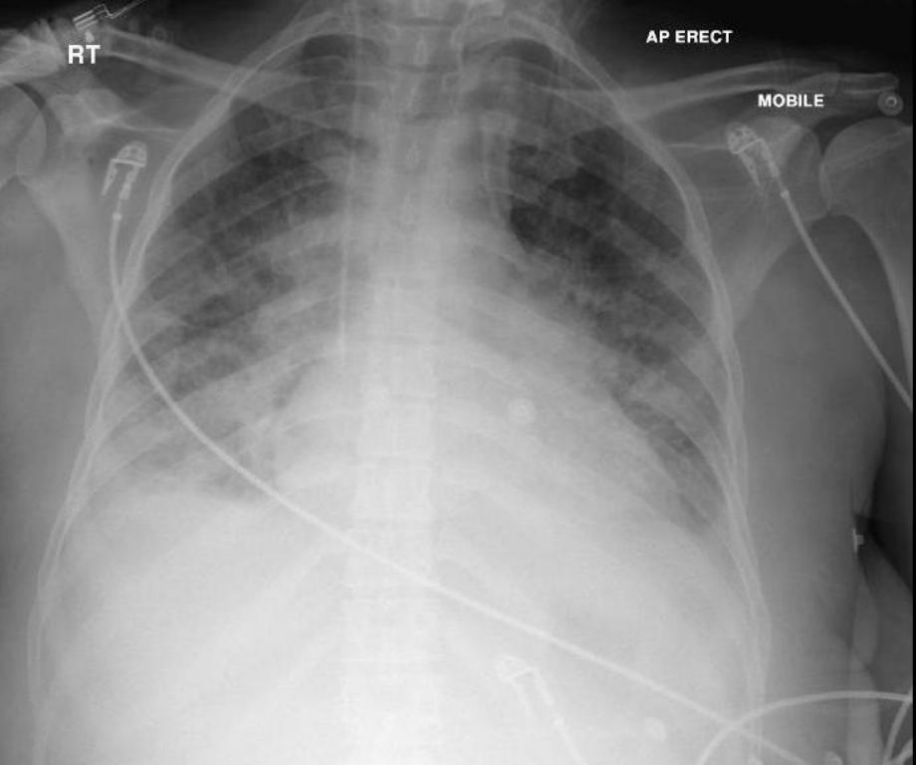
Chest X-ray showing bilateral diffuse infiltrates.
